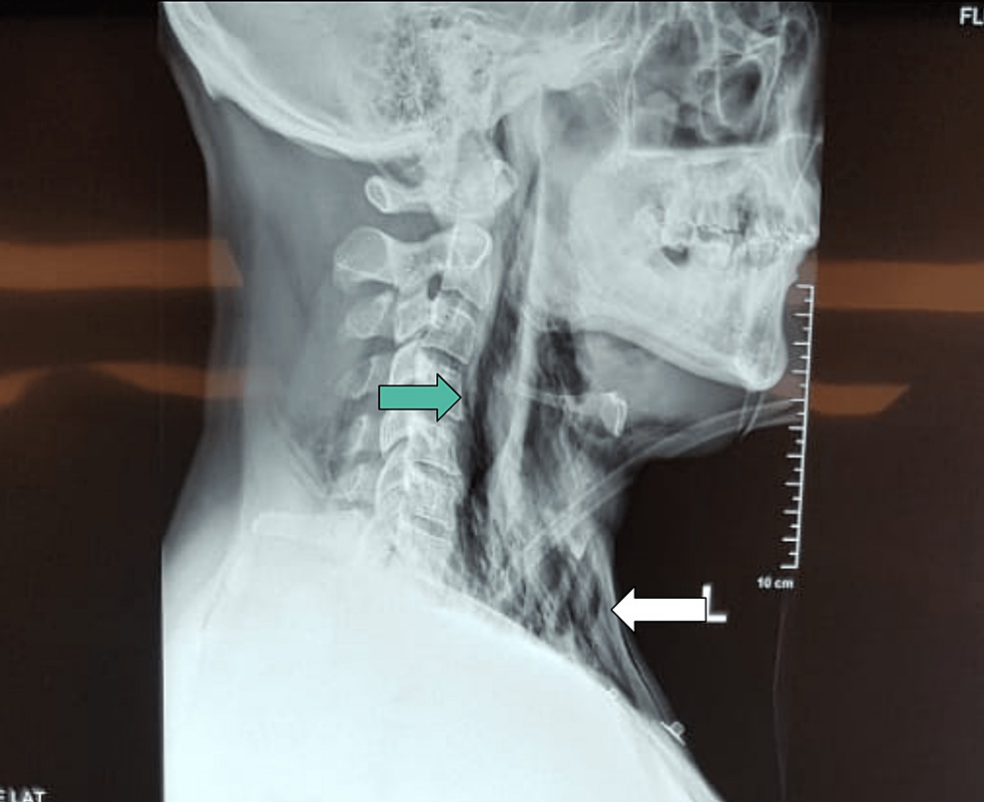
Lateral neck radiograph showing extension dissection of prevertebral soft tissue by air up to nasopharynx (green arrow) and air in anterior cervical tissue (white arrow).
